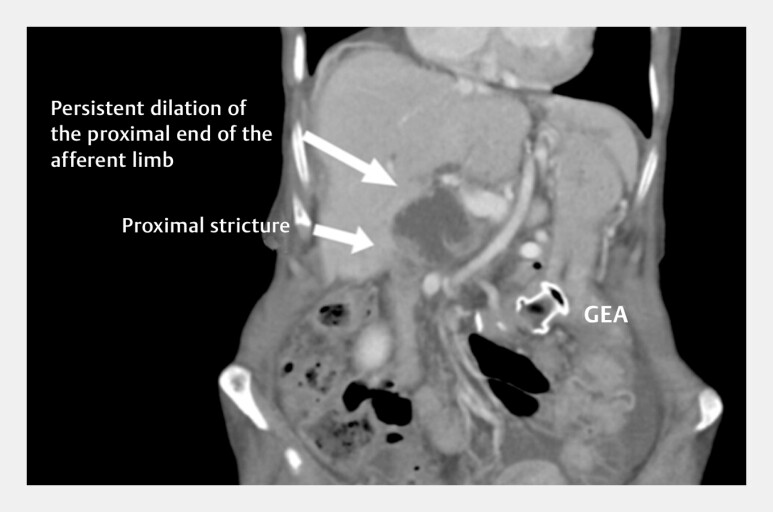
Persistent dilation of the biliary tree and the proximal end of the afferent limb, despite the technically successful placement of EUS-GE. EUS-GE, endoscopic ultrasound-guided gastroenterostomy.
